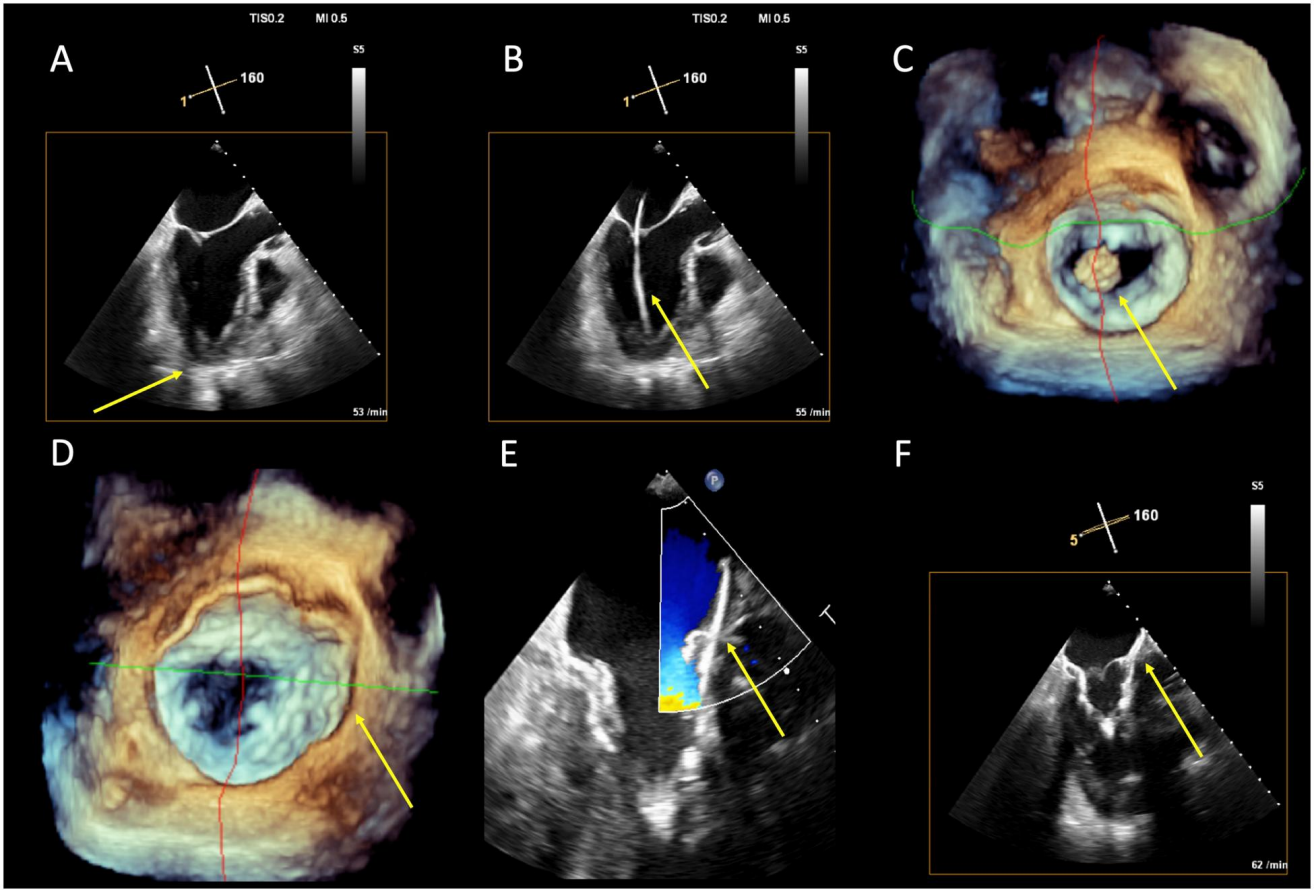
Procedural Steps and Echocardiographic Guiding of Transapical TMVI. (A) After exposure of the cardiac apex, transapical puncture is guided using bi-plane mode to display a bi-commissural and a 3-cavity (or LVOT) view. The arrow indicates the surgeon’s finger (poke test) in the 3-cavity view. (B) The implant catheter (arrow) is advanced over the standard guidewire through the valve and its position optimized using 2 reference views (here only 3-cavity shown), so that it is centred 1 to 2 cm above the mitral annulus. (C) The Tendyne valve (arrow) is mechanically advanced through the catheter under constant view. The prosthesis is exposed in the atrium and optimal rotation is confirmed. (D) The Tendyne valve is deployed and self-expands (arrow points at the outer border of the stent frame). (E) Once the prosthesis has been deployed, the valve is assessed using colour Doppler from 0 to 180° to detect PVLs and, if necessary, an increase in traction on the tensioner can be applied as a corrective manoeuvre. (F) in the 3-cavity view and in the apical trans-gastric view (5-cavity equivalent), the patency of the neo-LVOT (indicated by the arrow) is assessed and the tension reduced in the event of significant obstruction
